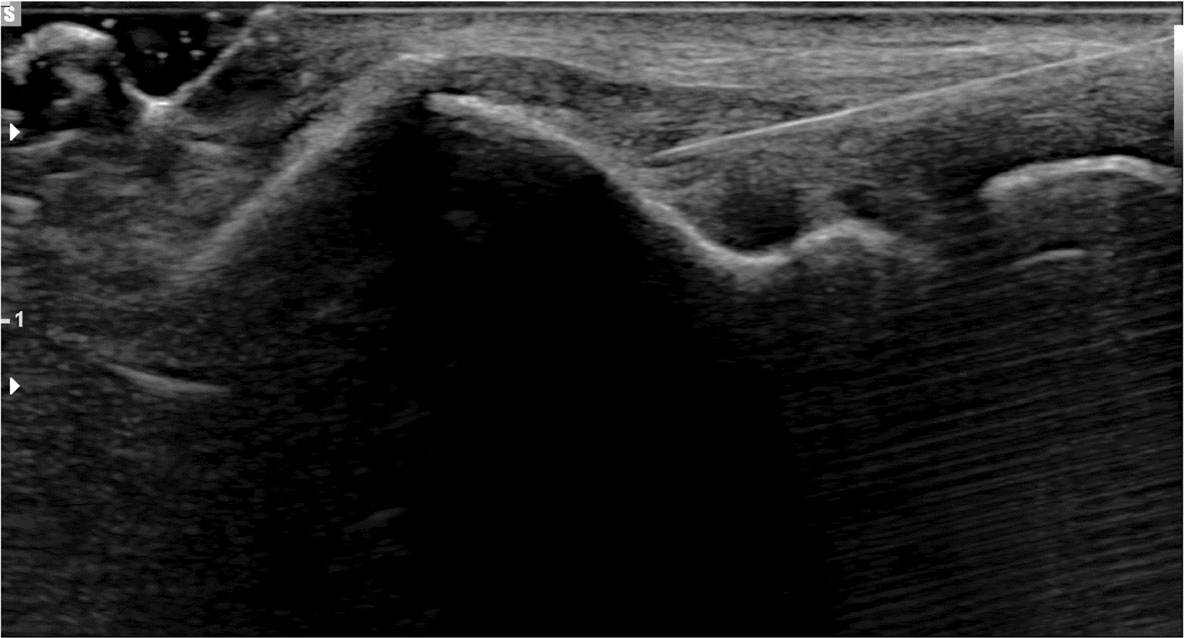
Longitudinal ultrasonogram of the Common Extensor Tendon (CET). The hypodermic needle can been seen entering at the right side of the image. This particular specimin underwent a 1 ml single shot injection
